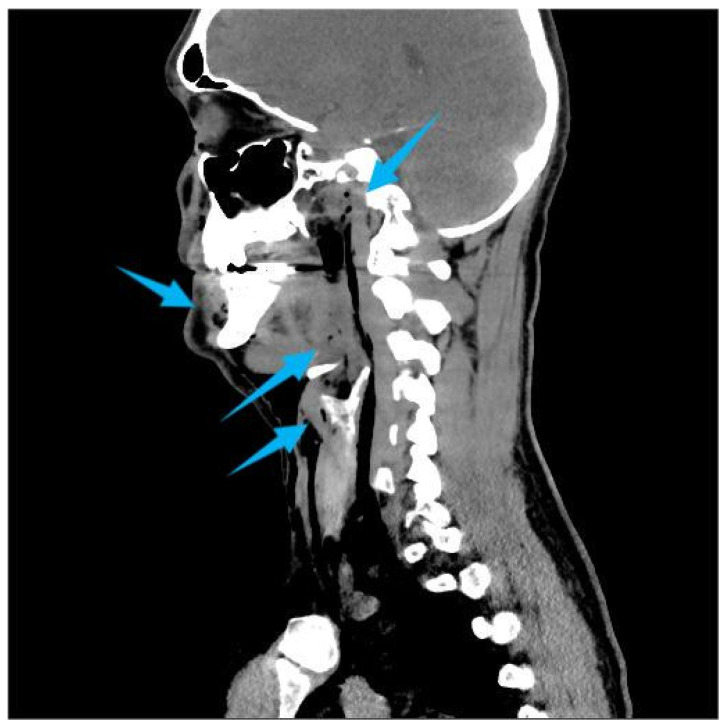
Sagittal Multislice Computed Tomography (MSCT) image demonstrating submucosal emphysema of the nasopharynx. The arrows highlight the presence of emphysema.
